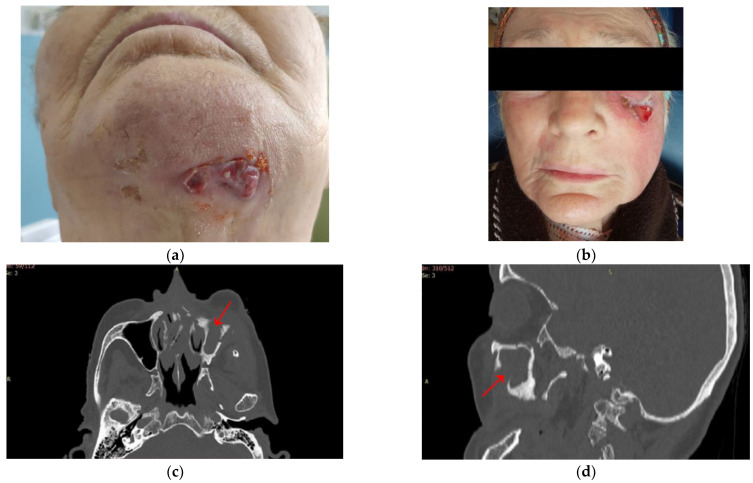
(a) Extraoral appearance. Female patient with high bone osteonecrosis in the submental region, with extensive infection. This infection has become evident through a chronic submandibular fistula. (b) Extraoral appearance. Female patient with high bone osteonecrosis in the left zygomatic region, with maxillary sinus involvement. This infection has become evident through a chronic facial fistula. (c) CT axial section showing opacification of the left maxillary sinus. (d) CT sagittal section showing an oro-antral communication on the left side and opacification of the left maxillary sinus.
